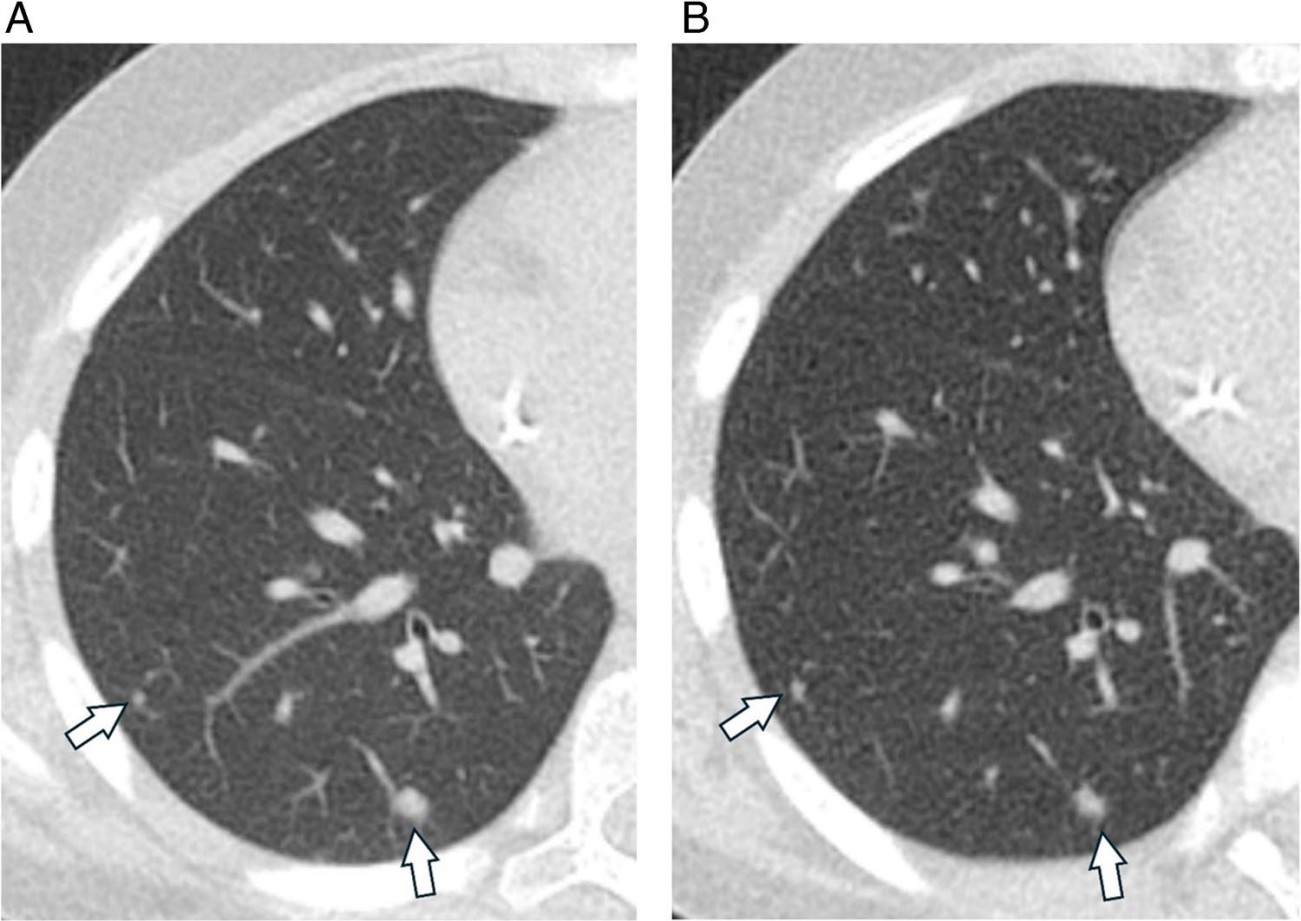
Clinical and reduced-dose CT images of an 11-year-old male with osteosarcoma. A clinical dose and B reduced dose axial CT images show two right lower lobe pulmonary nodules (*arrows*)
